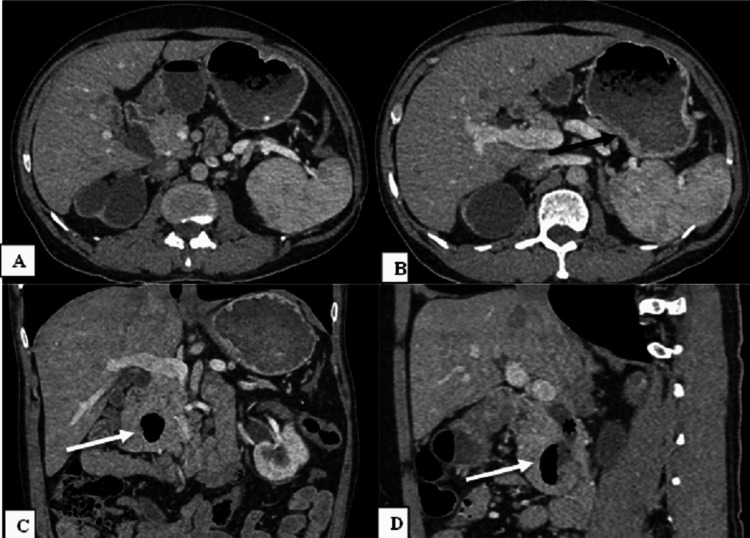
Partial dorsal agenesis of the pancreas. Axial contrast-enhanced computed tomography of the abdomen showing non-visualization of the body and tail of the pancreas (A) and dependent stomach sign (B, black arrow). Coronal contrast-enhanced computed tomography of the abdomen (C and D) showing Lemmel syndrome (dilatation of common bile duct (black star) due to duodenal diverticula (white arrows)).
